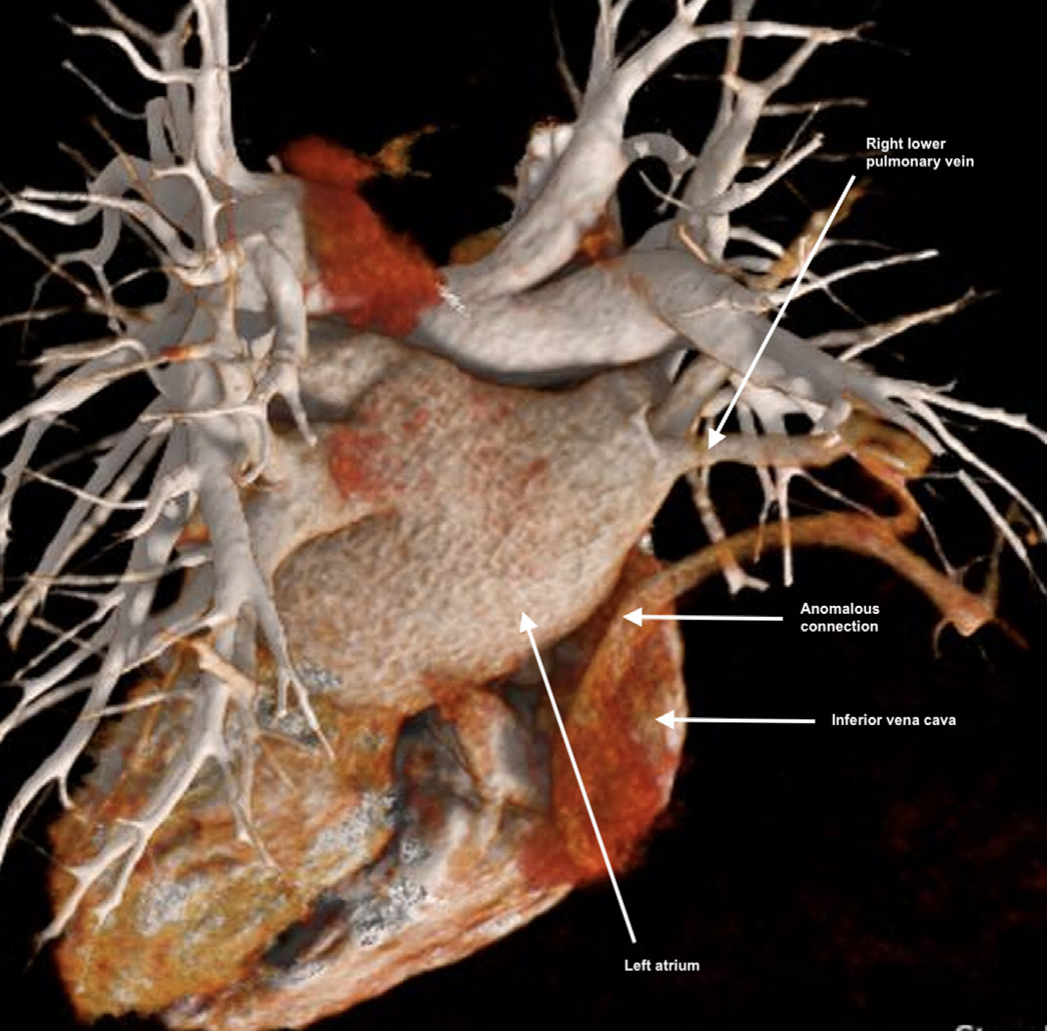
Anomalous Pulmonary Vein-Inferior Vena Cava Connection as Cause of Paradoxical Embolism Three-dimensional computed tomography reconstruction showing anomalous connection between the suprahepatic inferior vena cava and the right lower pulmonary vein.
